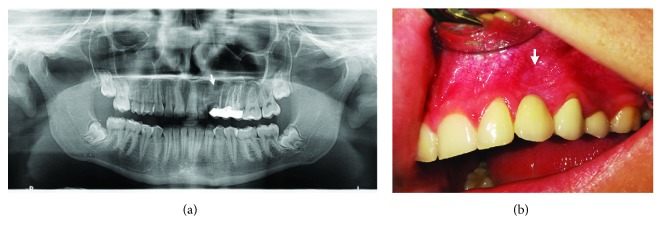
Follow-up period after 9 years. (a) An orthopantomogram (OPG) with no signs of radiolucency with satisfactory bone regeneration in the operated area (arrow). (b) Clinical appearance of vestibular mucosa with the presence of mild recess (arrow).
